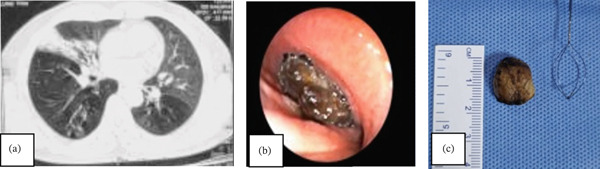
(a) Chest CT showing consolidation‐atelectasis of right middle lobe. (b) Deeply impacted FB at the origin of right main bronchus. (c) Retrieved FB (hog plum nut) with dormia basket.
